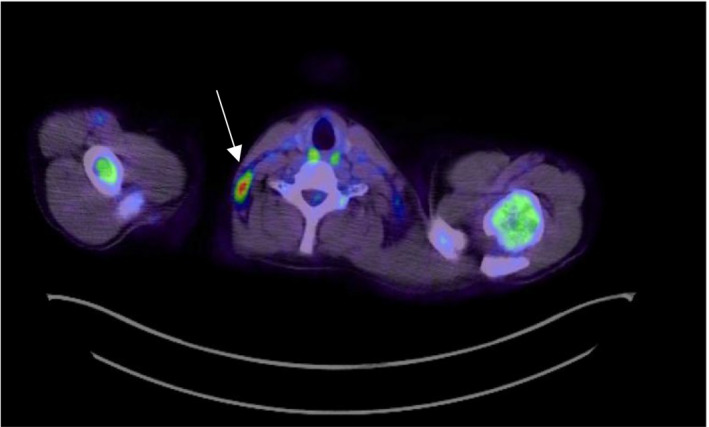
Hypermetabolic right cervical lymph node, 0.9 cm in size, SUV of 8.5. (White arrow)
